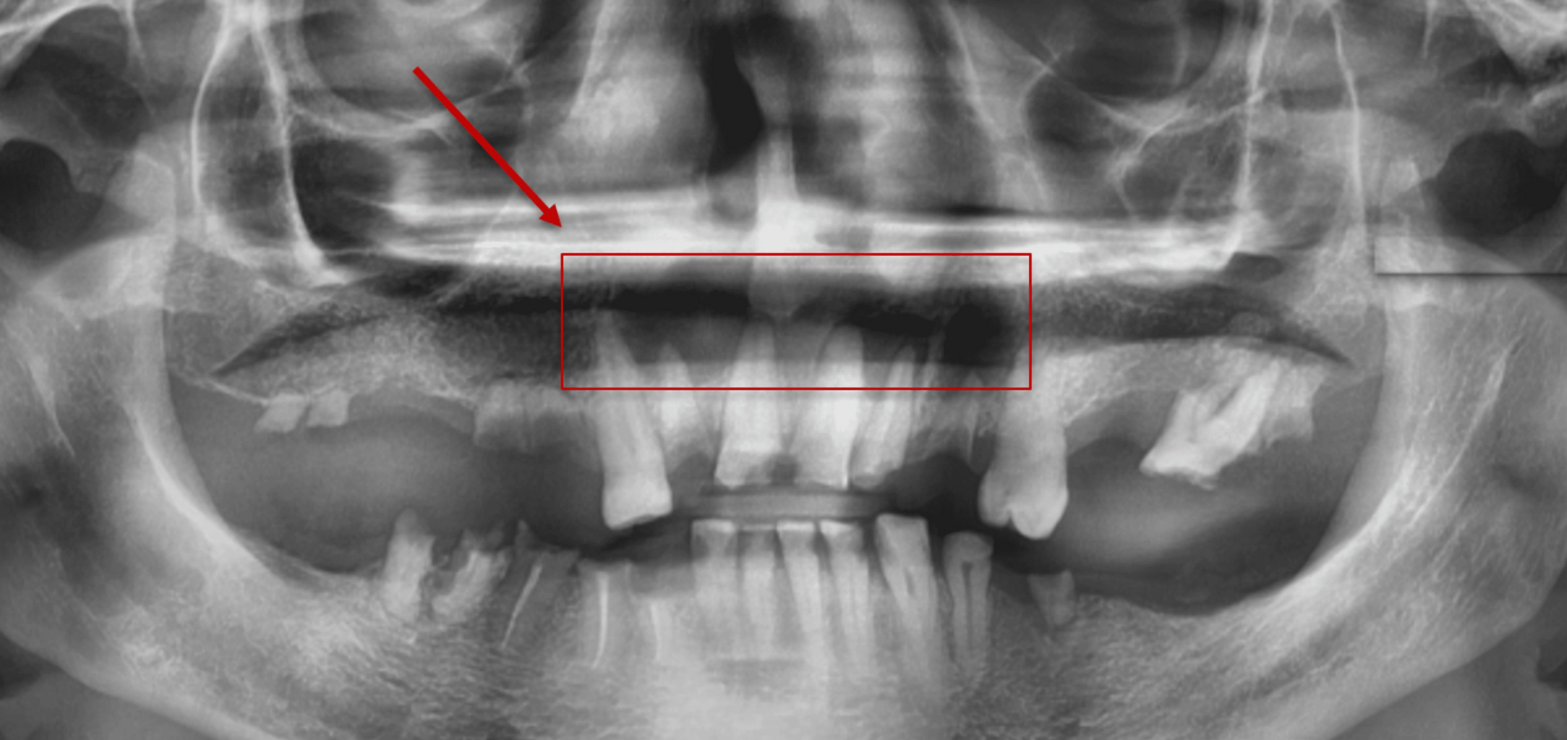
A panoramic radiograph of a 51-year-old male patient showing multiple periapical radiolucent lesions on both sides of the anterior maxillary region.
